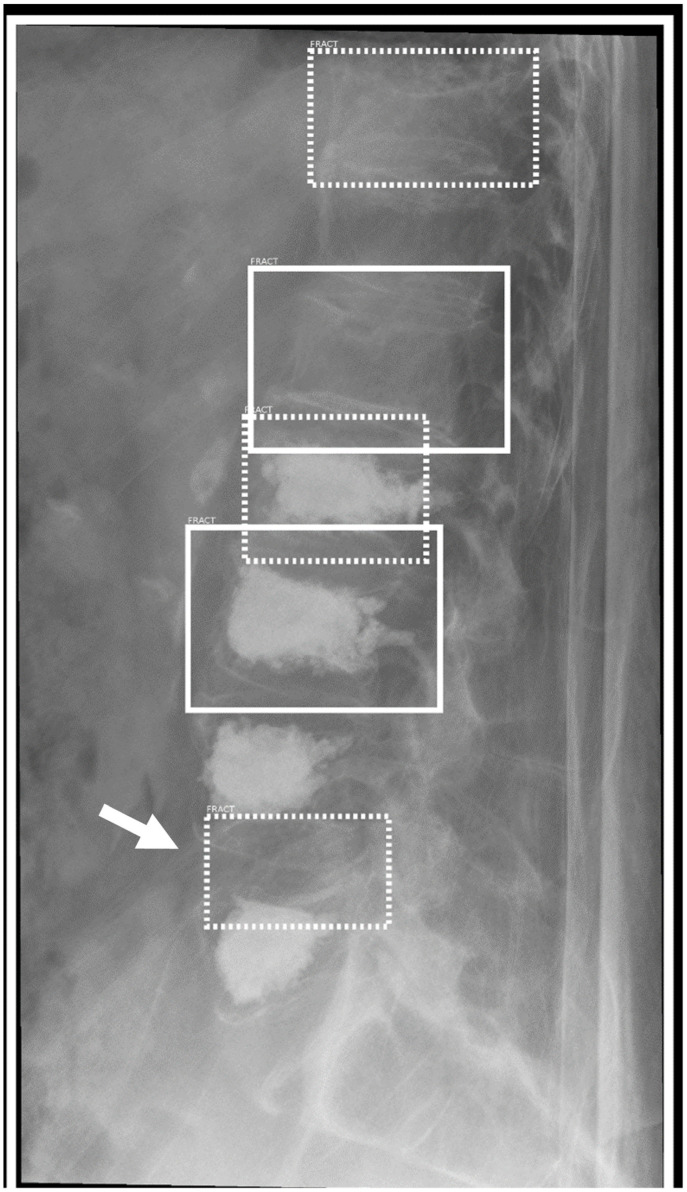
Lateral radiograph of the lumbar spine. Multiple (older) vertebral compression fractures are correctly marked as “Positive” (through line bounding box) and “Doubt” (dashed line bounding box). The bottom bounding box marks the intervertebral space L4/5 (arrow) an obvious mistake of the AI.
